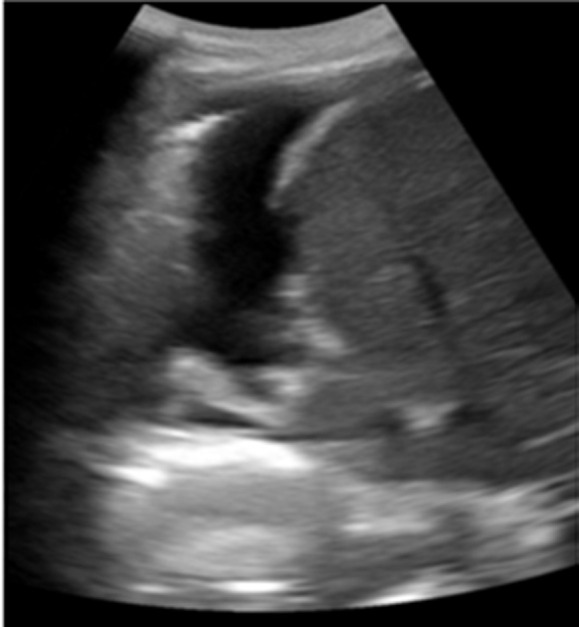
ultrasound showing minimal right-sided pleural effusion
